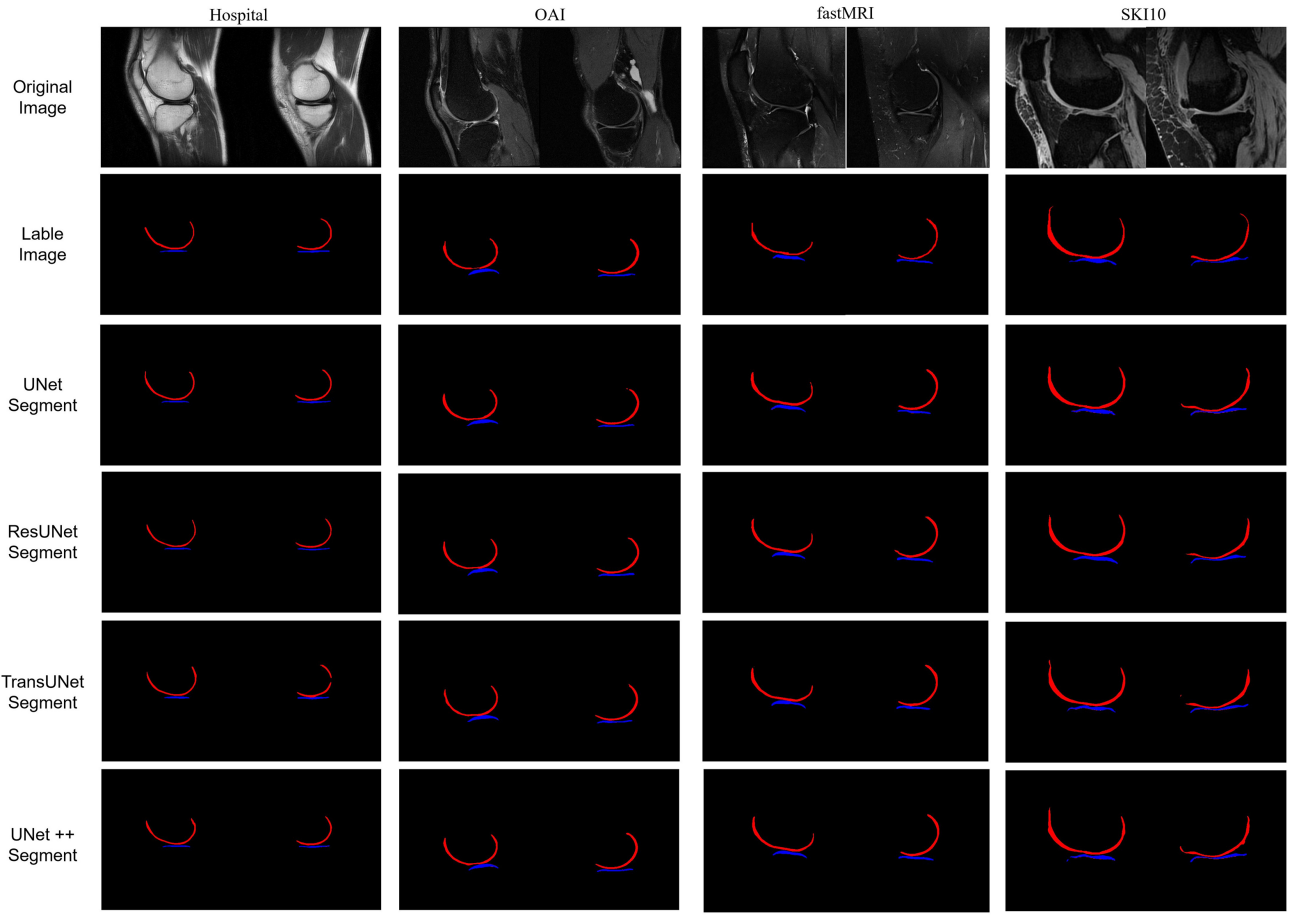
The first set represents hospital data; the second set represents OAI data; the third set represents fastMRI data; and the fourth set represents SKI10 data, which was pre-labeled. For each set, the first row displays the original MRI images of the knee joint’s medial and lateral sides. The second row shows the labeled cartilage images. The third to sixth rows depict the post-prediction images.
